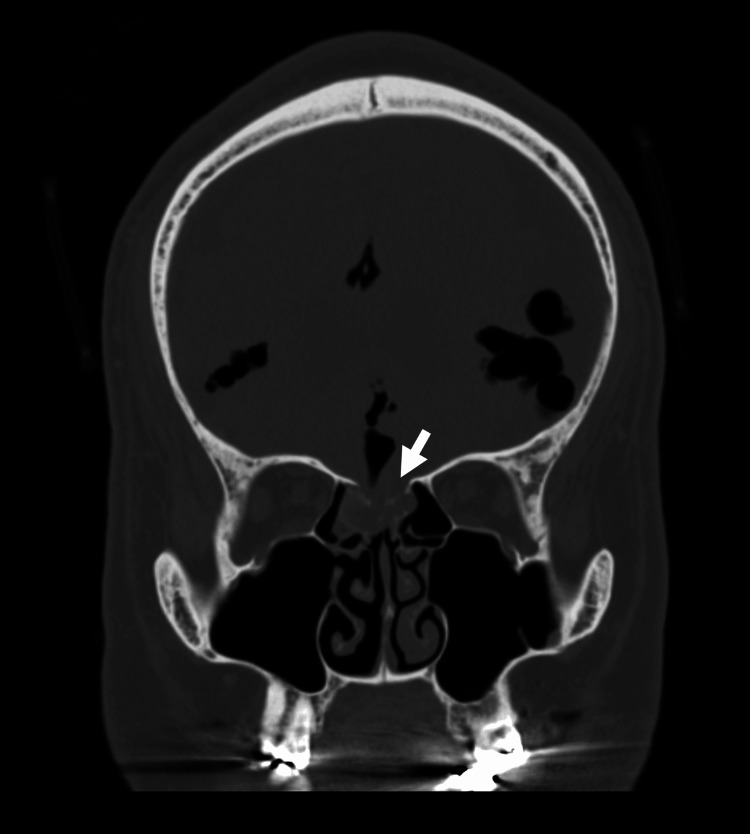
Computed tomography (CT) scan of the head upon admission, revealing a bony defect in the frontal cranial fossa with communication to the ethmoid sinus. The white arrow indicates a bone defect in the anterior cranial fossa.
